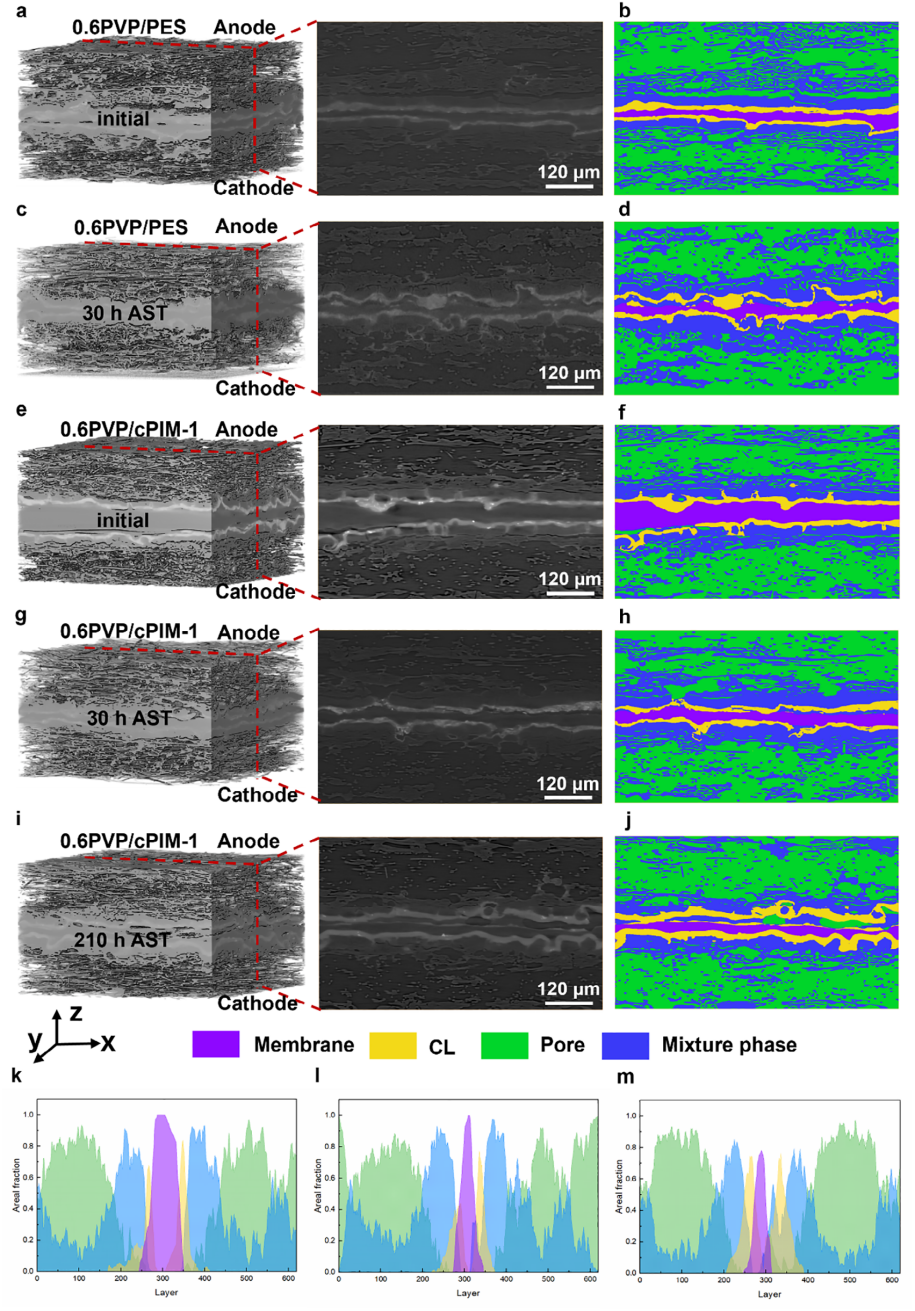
PA migration behavior in MEA. 3D X‐ray CT segmentation and the layer orthoslice of a,b) initial, c,d) 30 h AST 0.6PVP/PES and e,f) initial, g,h) 30 h AST, i,j) 210 h AST 0.6PVP/cPIM‐1. Slice‐by‐slice plots of area fraction in the Z‐direction of 0.6PVP/cPIM‐1 at k) initial, l) 30 h AST and m) 210 h AST state.
